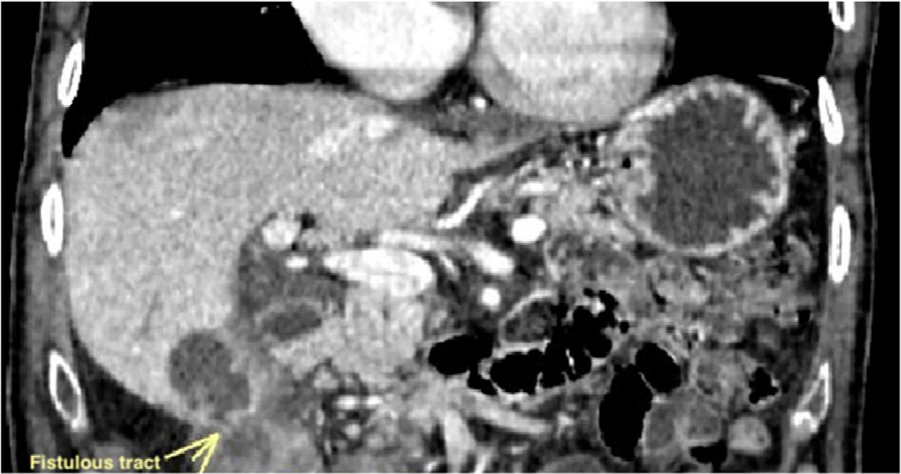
Coronal CT image shows a close approximation of hepatic flexure of colon with the gallbladder which suggests a fistulous communication (arrow).
